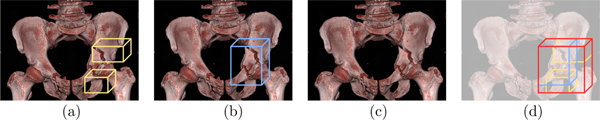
An example of 3D fracture fusion in augmentation-based self-ensembling inference. Figure (a), (b), (c) are 3 augmented CT scans. Boxes in (a) and (b) are 3D fractures fused from Faster RCNN results and are of the same fracture type. Red box in (d) is the final fused 3D fracture. Because fracture in (b) is intersected with both fractures in (a), they are fused and the red box in (d) is the final fused fracture. As the result, augmentation-based self-ensembling inference mitigates the 2D mis-detection (between the 2 yellow boxes in axial direction) in figure (a). The confidence score is the frequency score of fractures among all 3 augmented CT scans, which is 0.667.
